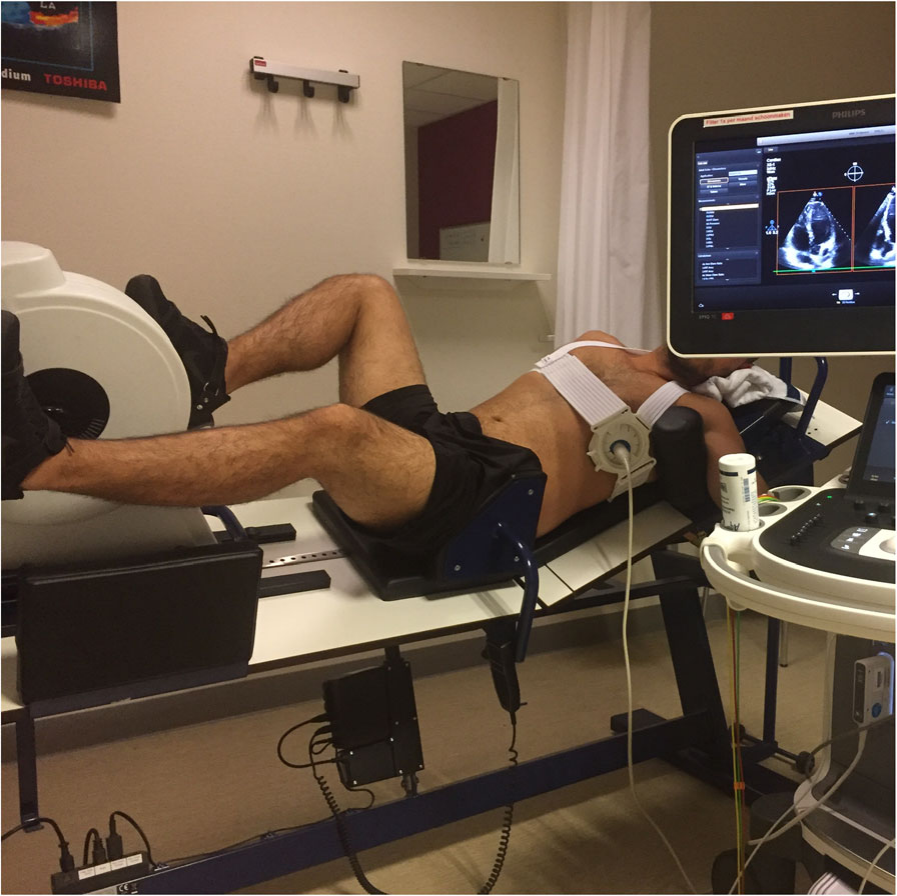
Supine bicycle ergometer test with stress echocardiography. Example of stress echocardiography with a patient lying on a supine bicycle ergometer. The table is tilted towards the left, to optimize the image quality of the apical four chamber view. The probe (X5-1, Philips Medical Systems, Best, The Netherlands) is fixated with Probefix and angulated in the optimal position. See the online movie for an overview of the supine bicycle test with echocardiography using the Probefix (Additional file 2)
